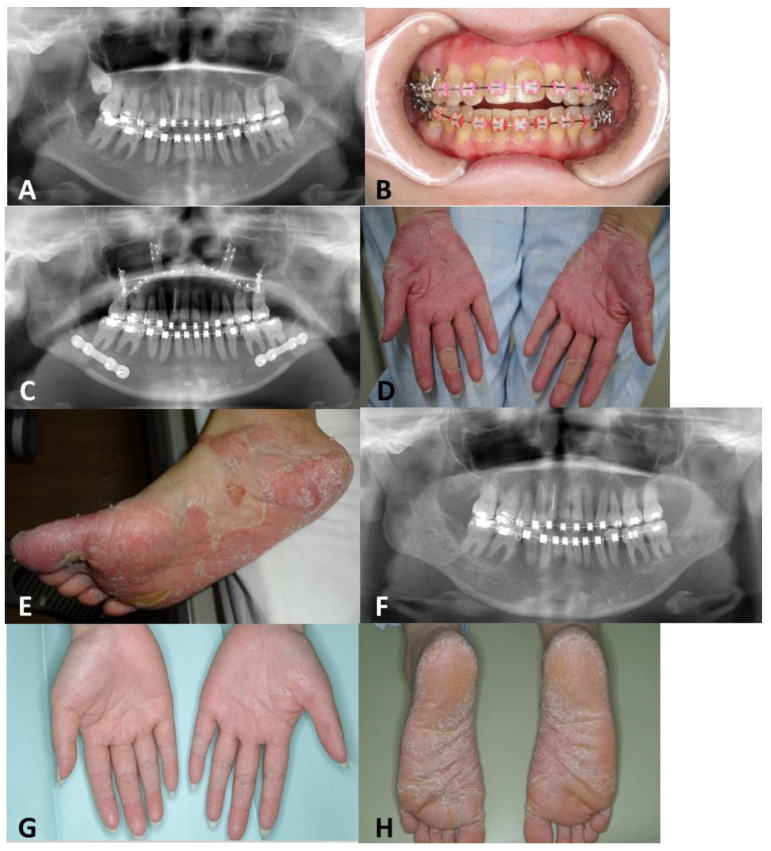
Preoperative and postoperative panoramic X-ray and photographs of palms and soles. (A,B) Preoperative panoramic X-ray and intraoral photographs are shown. (C) Osteotomies of the maxilla and mandible were performed and fixed with titanium plates and screws. (D,E) Symptoms of PPP worsened on the palms and soles. (F) Titanium plates were removed and re-fixed with absorbable plates. (G,H) After plate replacement surgery, the dermatologic symptoms on her palms and soles improved.
